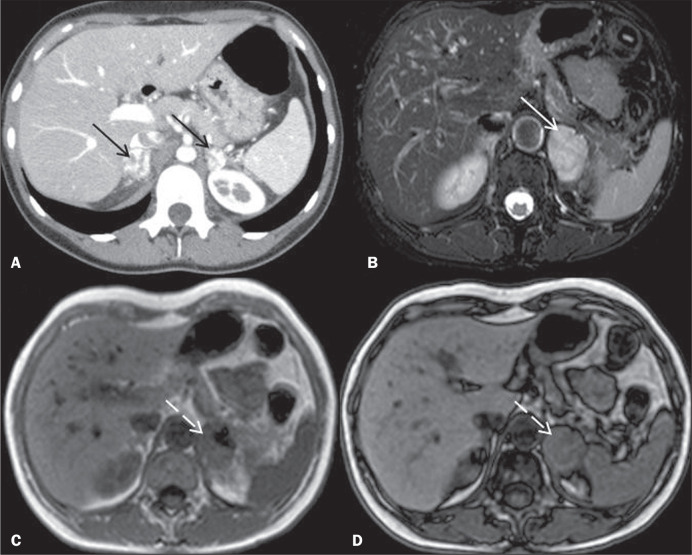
Contrast-enhanced axial CT scan in the arterial phase (A), showing
